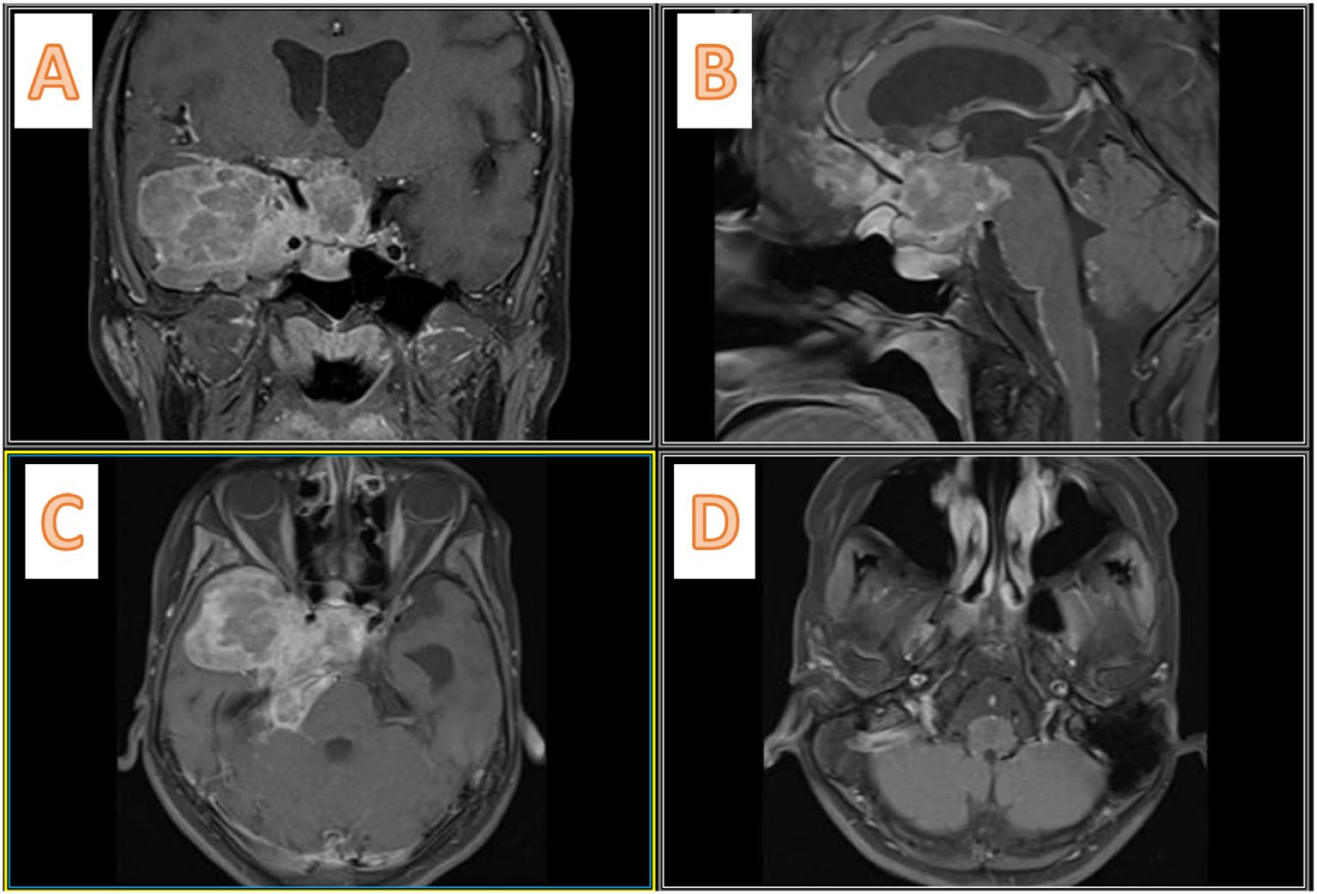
Preoperative Gadolinium‐enhanced MRI. (A) Coronal cut indicates heterogeneously enhancing lesion in sella turcica with Knosp Grade 4 on the right side and encasement of the carotid bifurcation. (B) Sagittal cut shows fine nodular enhancement over the leptomeninges of the brainstem, along the anterior cerebral artery, and over the medial surface of the frontal lobe. (C, D) The lesion extends through the tentorium cerebelli to the posterior fossa; as depicted in (D), there is leptomeningeal enhancement over the vestibulocochlear complex.
